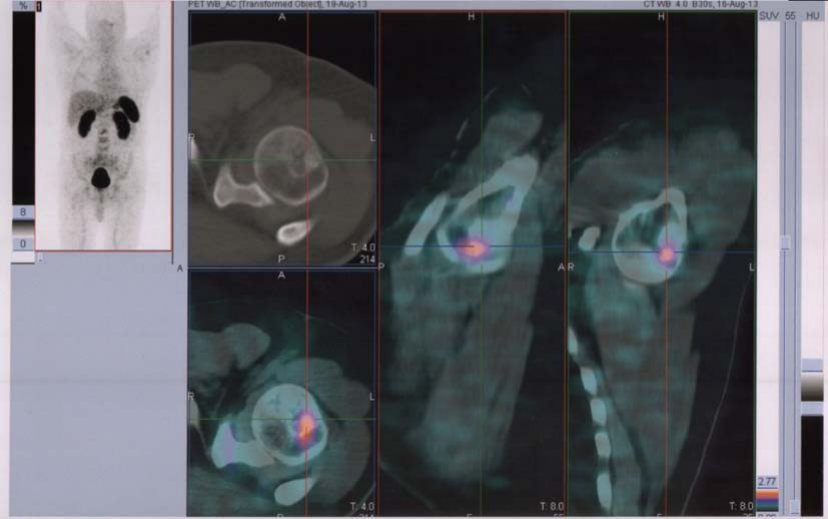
^68^Ga-DOTANOC transaxial PET/CT at the level of left humeral head showing increased uptake of the radiopharmaceutical in a mixed metastasis (pointers).
